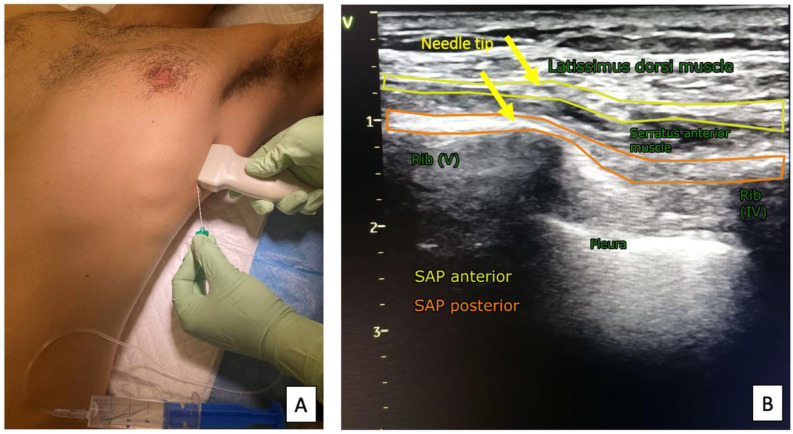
Ultrasound transducer positioning (A) and sonoanatomy of the SAP block (B).
